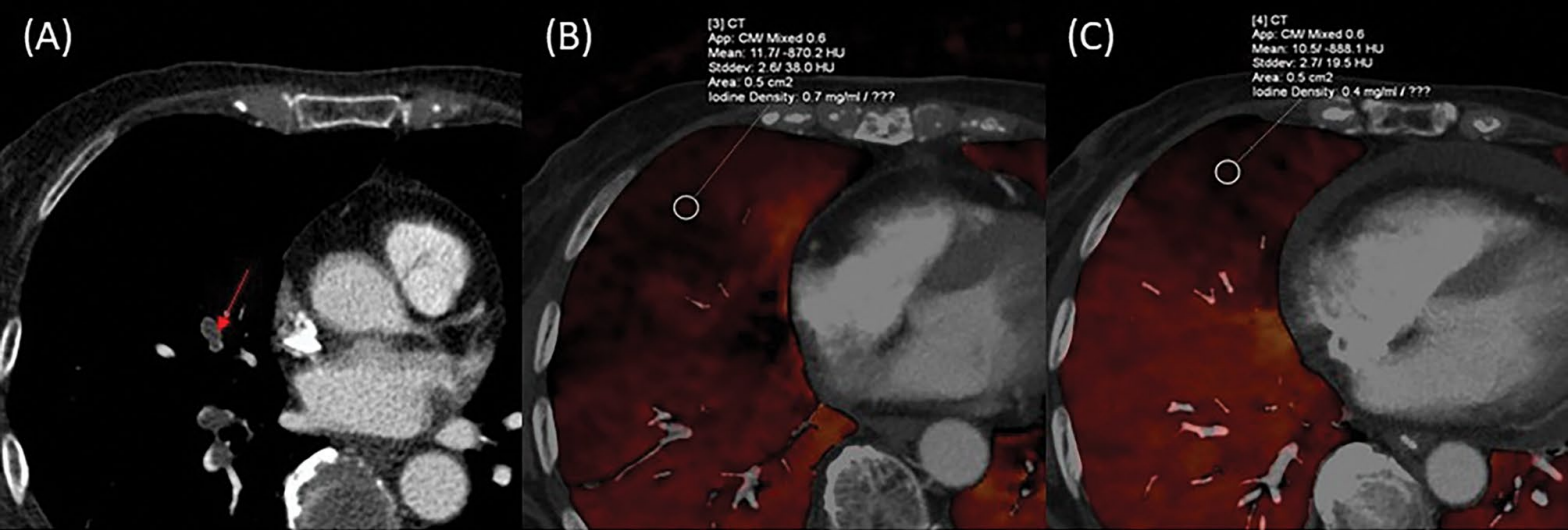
A 68-year-old man with pulmonary embolism. (A) Pre-treatment computed tomography pulmonary angiography indicates central occlusive thrombus from the right middle lobar pulmonary artery to A5. (B) Pre-treatment perfused blood volume shows perfusion decrease in right S5. The mean CT value of the iodine map is 11.7 HUs. (C) Lung perfusion blood volume remains decreased even after treatment. Mean CT value of the segment is 10.5 HUs.
